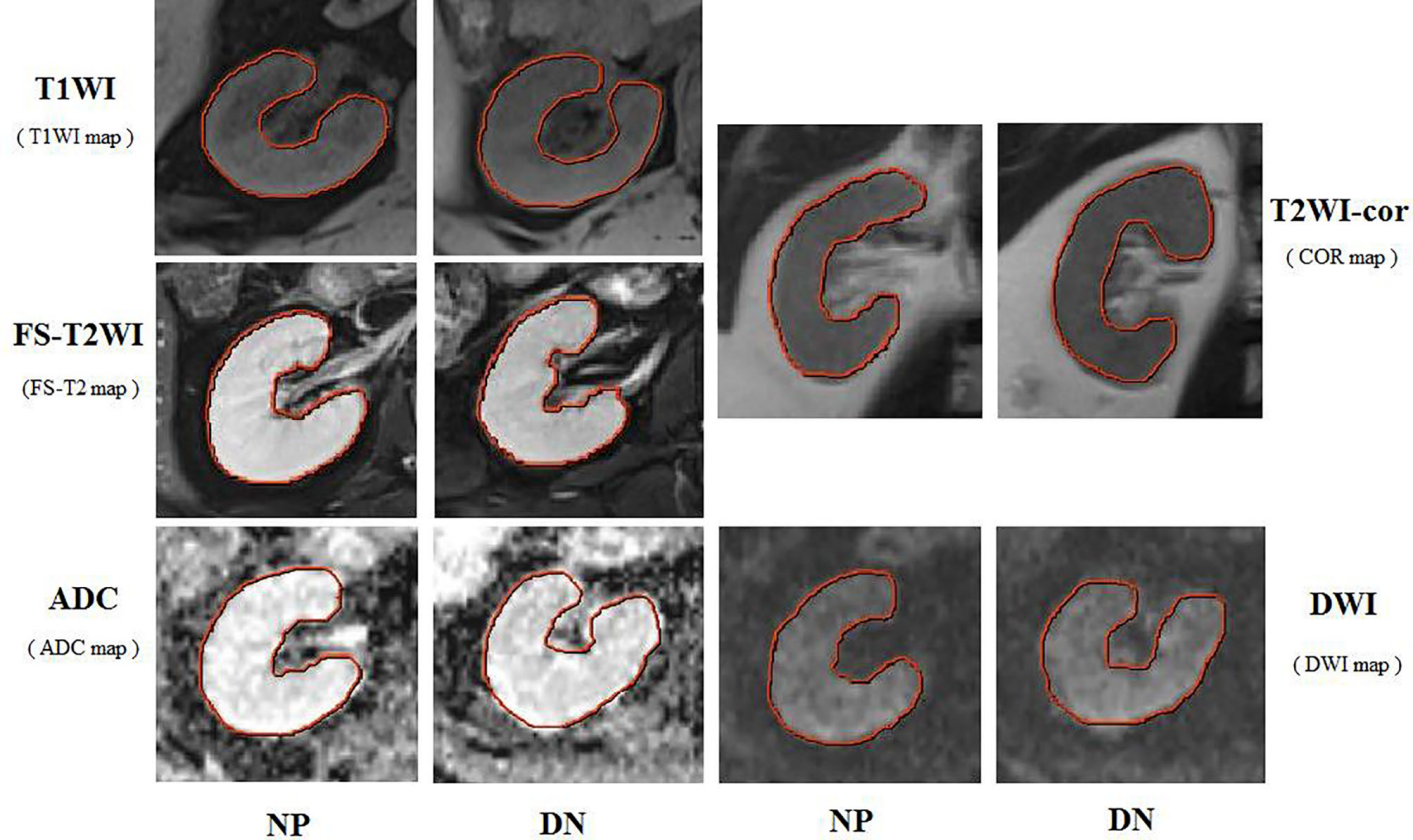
Respective MRI images of the right kidney with stage III type 2 DN and normal persons (NP). The ROI were curved over the renal parenchyma of the right kidney (red curve). In this study, the images analyzed were the T1WI maps, FS-T2WI and T2WI-cor maps, DWI and ADC maps.
